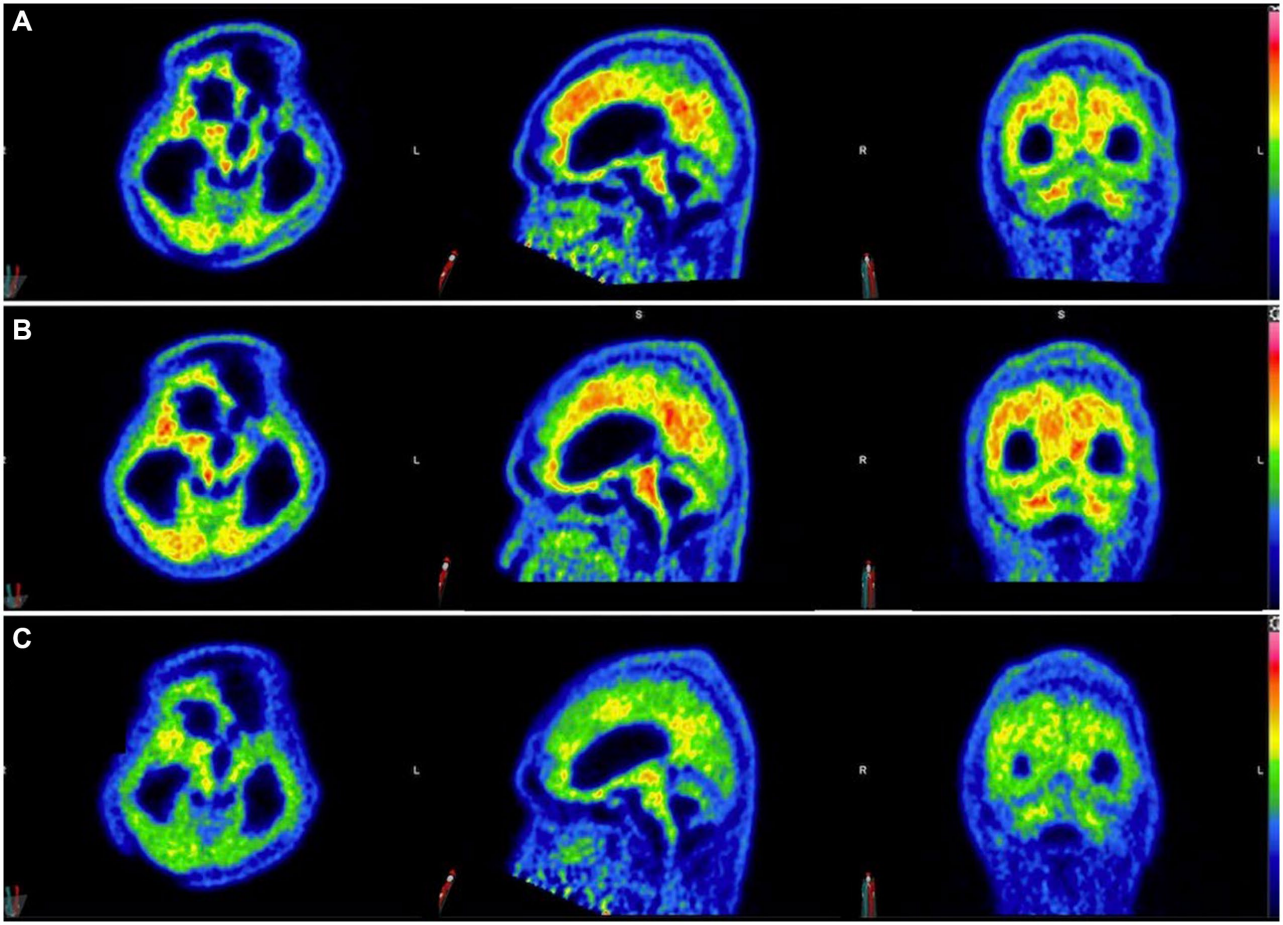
Serial ^11^C-Pittsburgh compound B positive emission tomography (^11^C-PiB-PET) scans following taxifolin use. Serial ^11^C-PiB-PET scans in axial, sagittal, and coronal views followed in response to taxifolin use after 6 months (A), 10 months (B), and 15 months (C), respectively. Visually, amyloid deposition can be gaged according to the colored bar on the right, which corresponds to the cortical-to-cerebellar standardized uptake volume ratio (SUVR; red = high uptake, yellow-green = moderate uptake, blue = least uptake). Note that the semi-quantification parameter cortical-to-cerebellum SUVR is equal to “SUV_mean_/SUV_mean_ in cerebellum,” where SUV_mean_ is the mean SUV within each cortical volume of interest.
